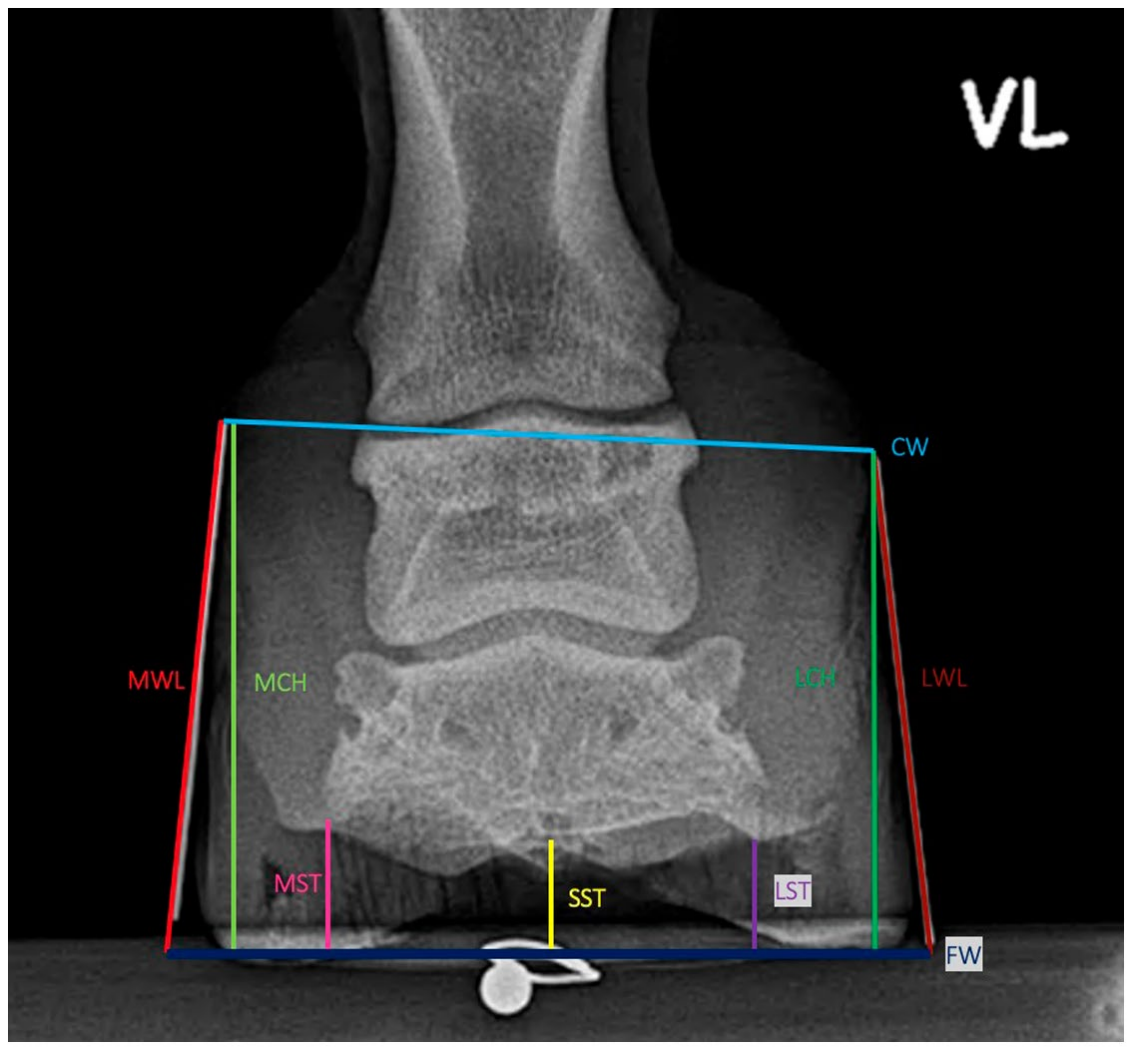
Length measurements on the dorsopalmar X-Ray.
